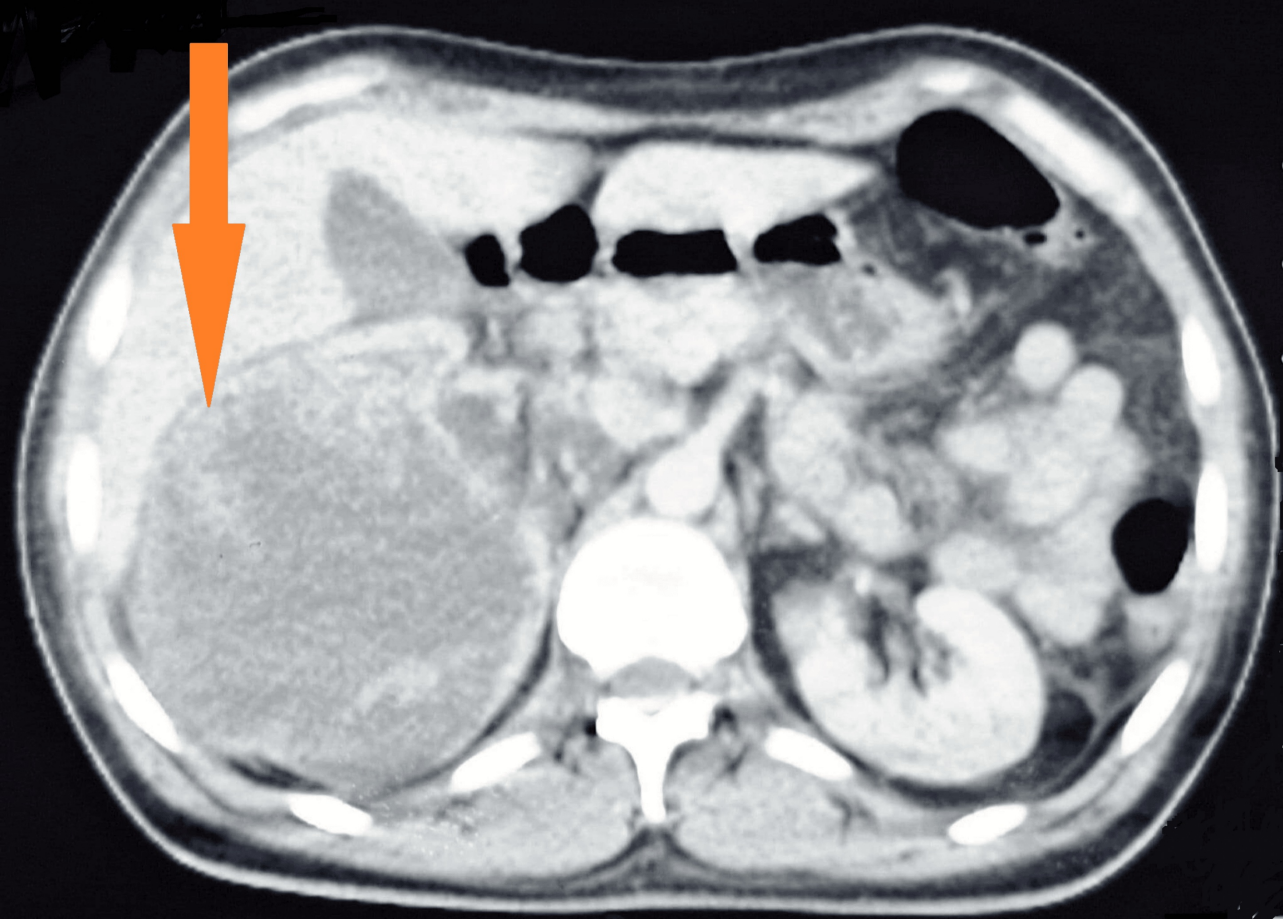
Axial CT scan image (arrow). The CT scan image shows a large right renal mass (arrow) with massive fluid containing thick partitions and a tissue portion.
